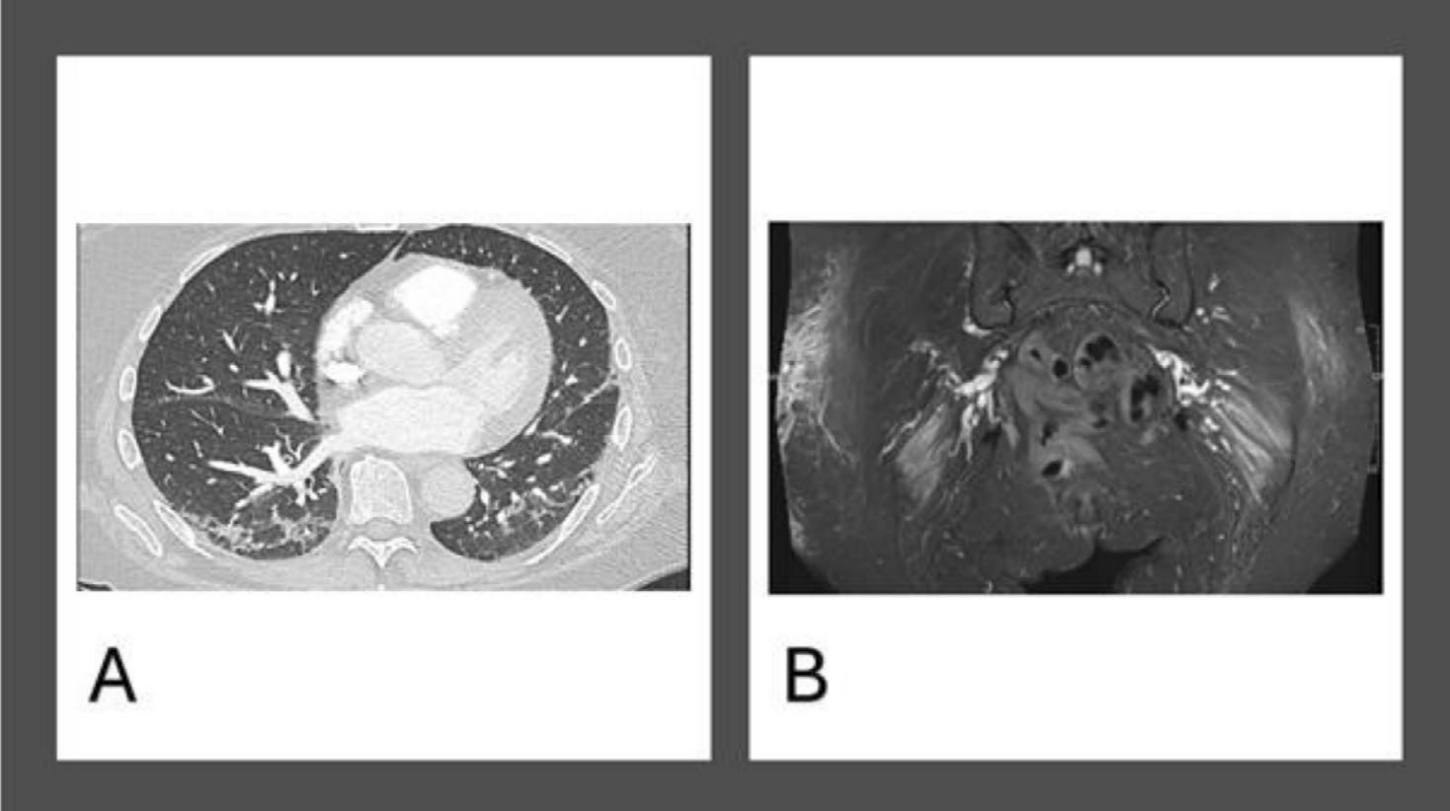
(A) CT chest imaging of the patient showing bilateral basal peripheral and perilobular consolidations. (B) An MRI of both femurs demonstrating diffuse asymmetric increased fluid signal on STIR involving the gluteus maximus muscles bilaterally, slightly more on the left and subcutaneous oedema focused on the area of her Holster's sign.
